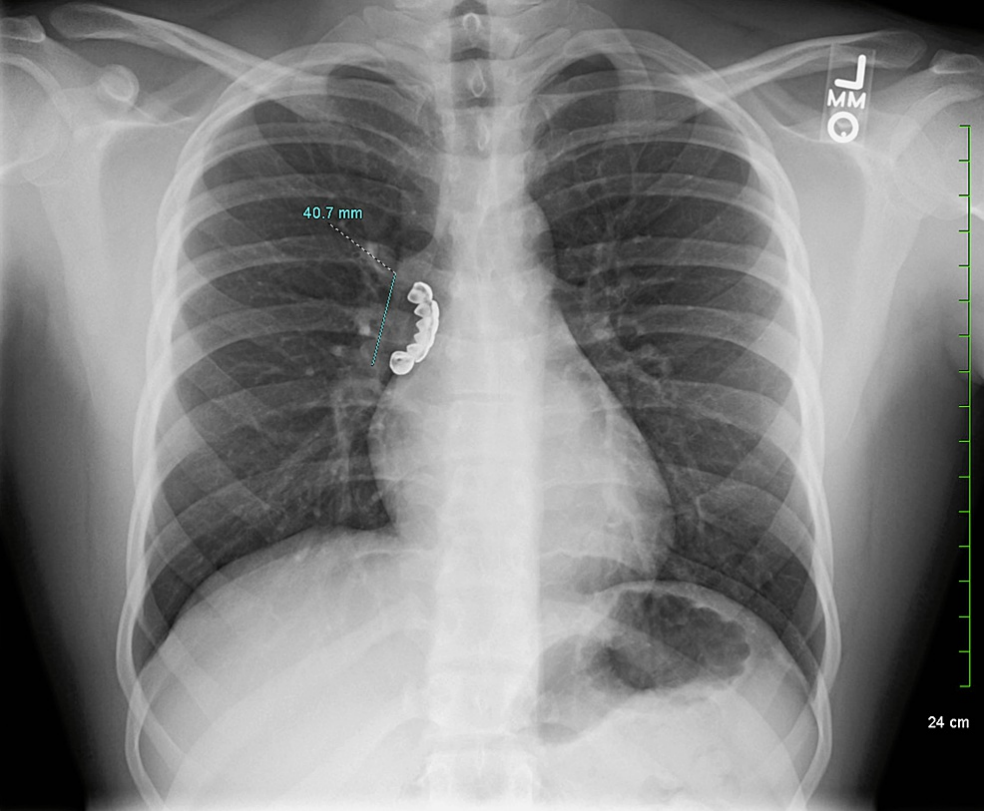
Initial chest radiography
